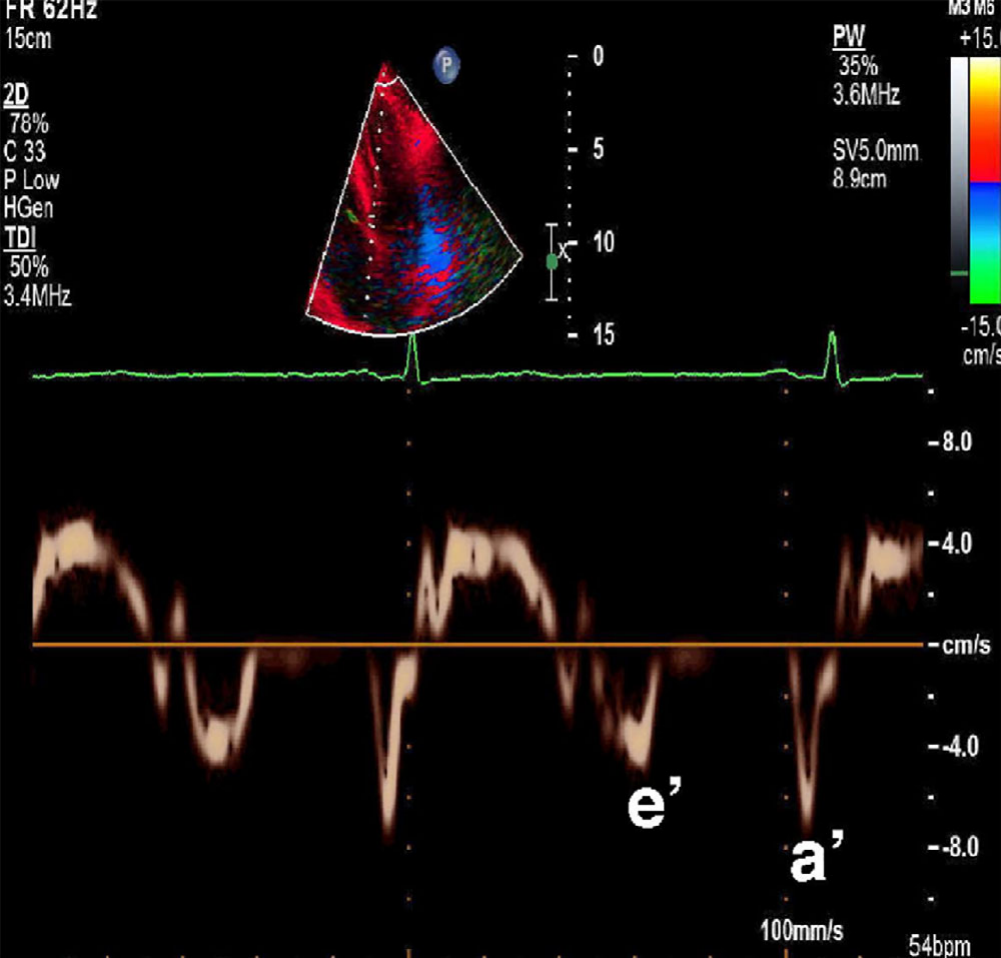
Tissue Doppler imaging (TDI) of the mitral annulus in restrictive cardiomyopathy. The spectral TDI tracing demonstrates a markedly reduced early diastolic mitral annular velocity (e′) and a relatively preserved atrial velocity (a′), consistent with impaired myocardial relaxation. The calculated E/e′ ratio is elevated (18), indicating elevated left ventricular filling pressures, a hallmark of restrictive physiology.
